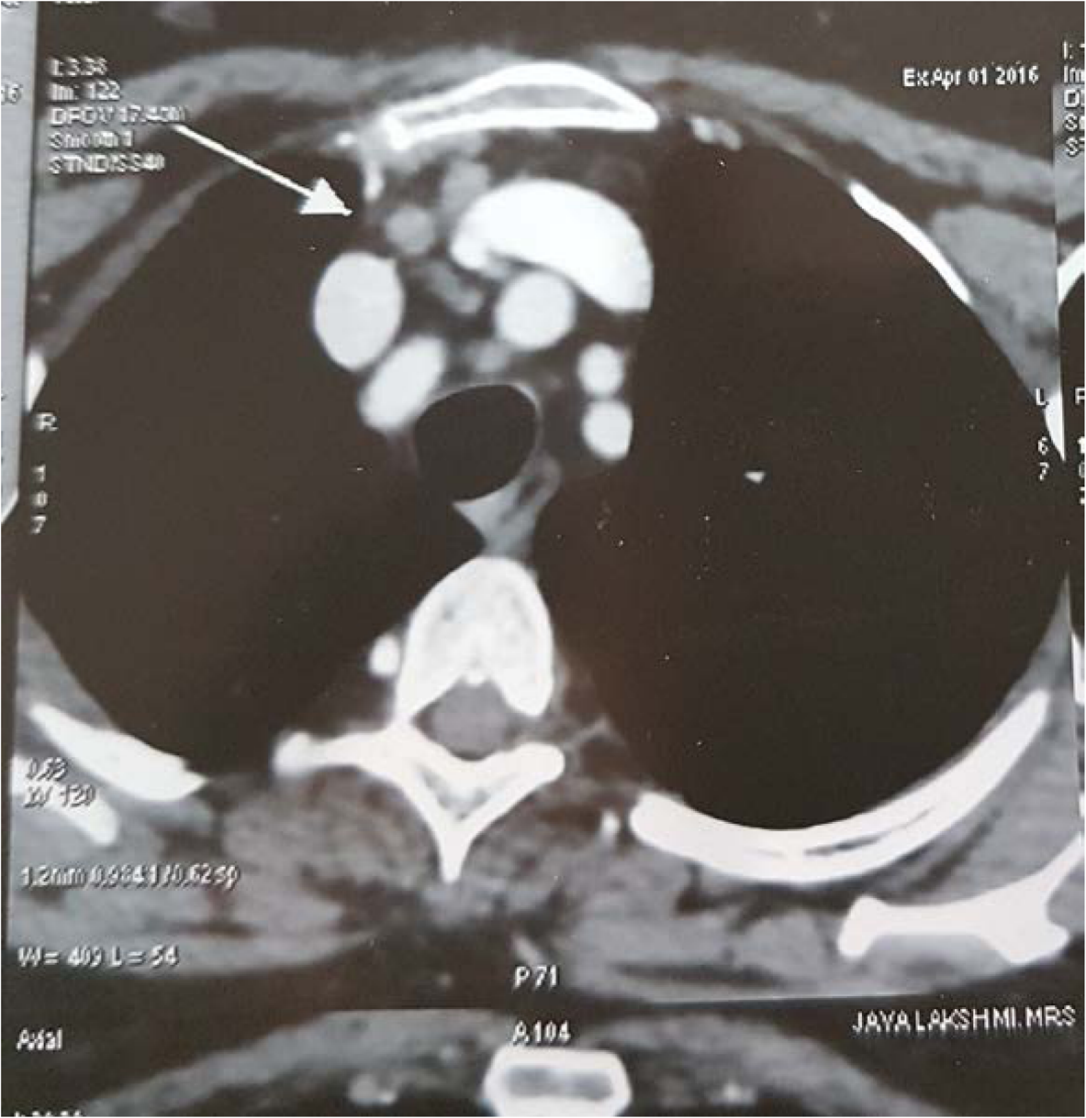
Plain HRCT chest showing calcified and non-calcified lung nodules
